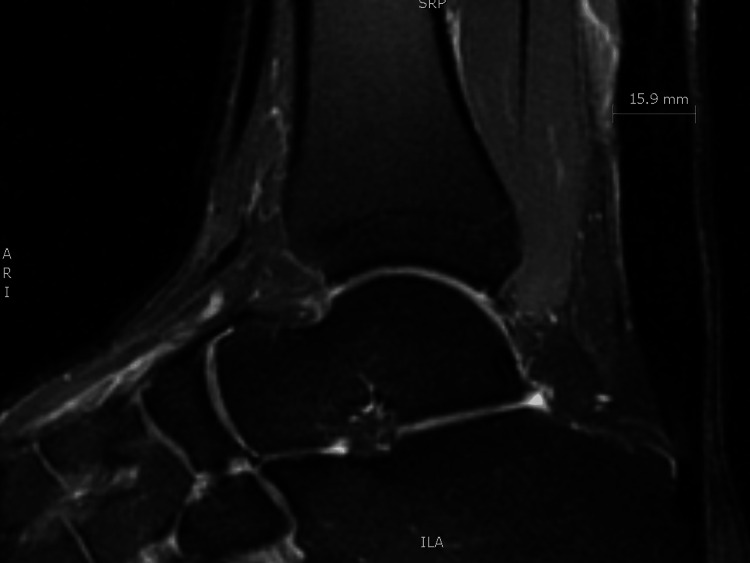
Ankle MRI of Patient 1 with T2 fat suppression (PD FS) sagittal image showing thickening of the Achilles tendon.
